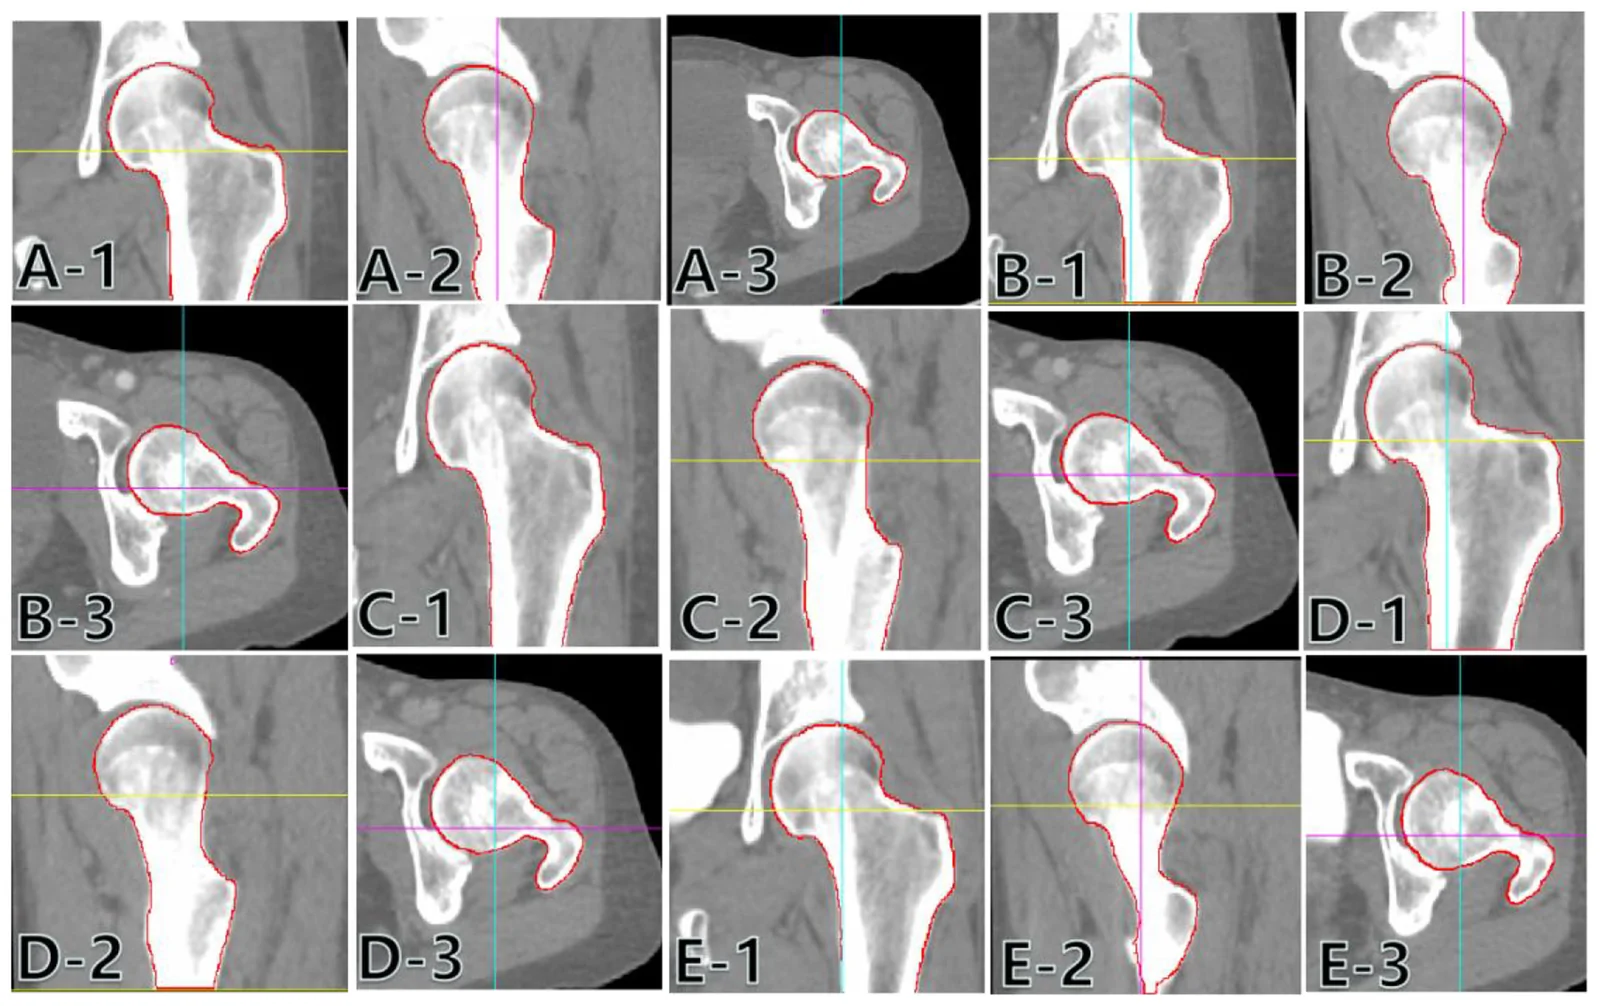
Representative BMD and *T*-value measurements in a 46-year-old female patient. (A-1, A-2, A-3) Plain (unenhanced) CT: *T*-value = −1.115; BMD = 359.27 mg/cm^3^. (B-1, B-2, B-3) Arterial phase: *T*-value = −1.066; BMD = 361.68 mg/cm^3^. (C-1, C-2, C-3) Venous phase: *T*-value = −1.040; BMD = 360.00 mg/cm^3^. (D-1, D-2, D-3) Delayed (late venous) phase: *T*-value = −1.016; BMD = 364.18 mg/cm^3^. (E-1, E-2, E-3) Excretory phase: *T*-value = −1.112; BMD = 359.39 mg/cm^3^.
